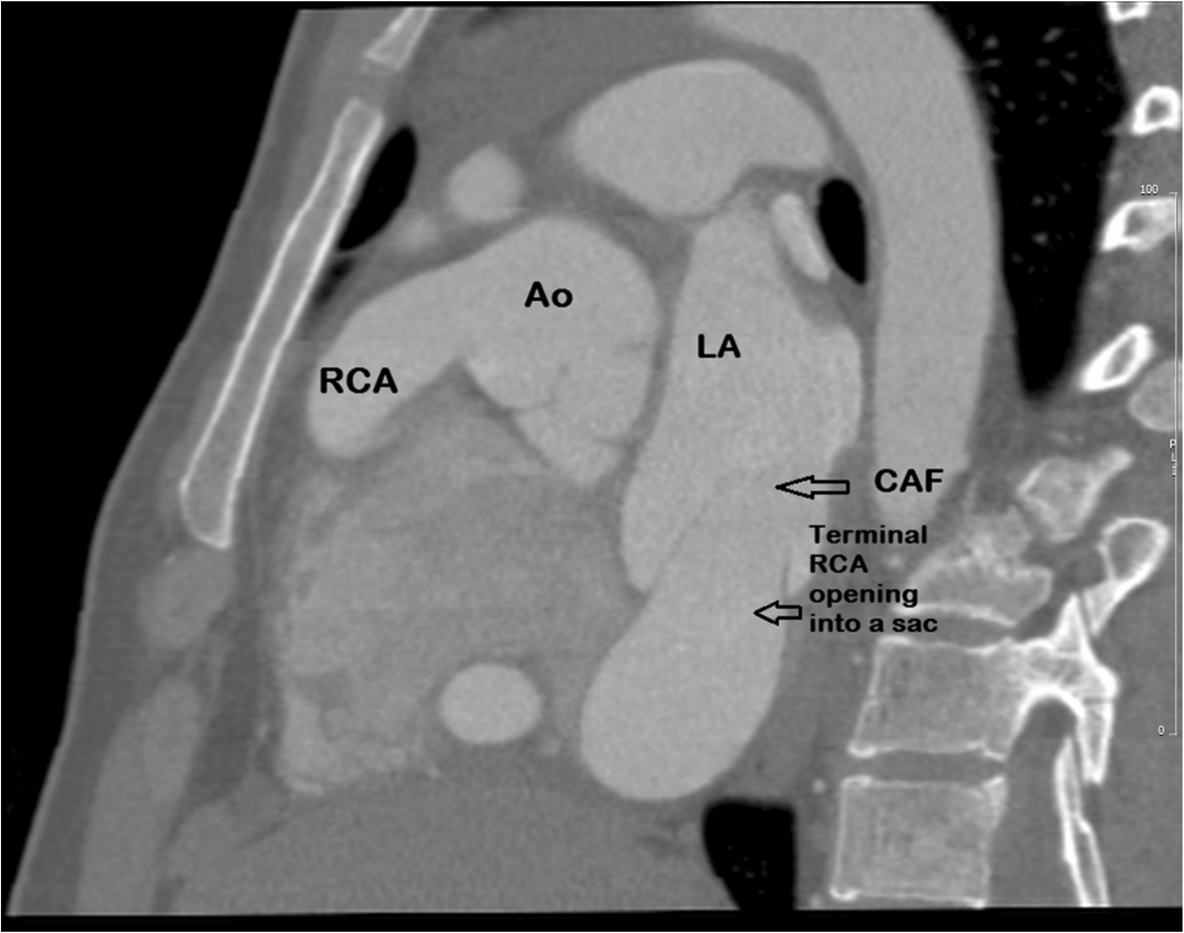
A CT angiogram (sagittal view) showing origin of the dilated coronary artery from the ascending aorta. Ao- Ascending aorta, RCA-Right coronary artery, LA-Left atrium, CAF- Coronary artery fistula
